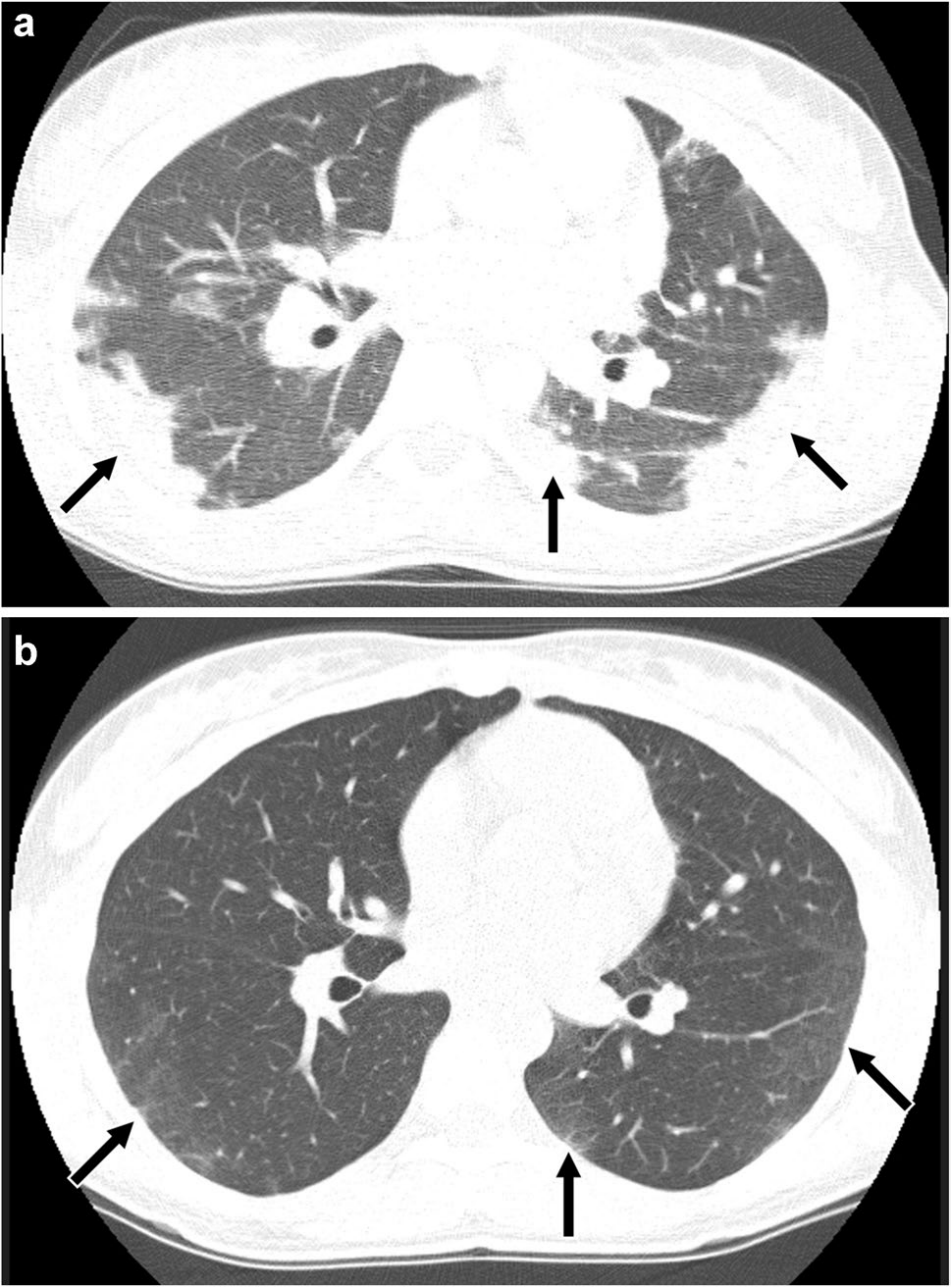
An 18-year-old female with a 3-week history of fever, cough, and dyspnea while receiving minocycline for acne. a Axial CT image with contrast showing bilateral peripheral consolidation (*black arrows*) typical of chronic eosinophilic pneumonia. b Axial image from follow-up CT with contrast obtained after medication discontinuation and 2 weeks of steroid therapy showing substantial improvement, with only mild patchy peripheral ground-glass opacity (*black arrows*) remaining at prior sites of consolidation consistent with minocycline-induced lung disease. (Images reprinted from Caffey’s Pediatric Diagnostic Imaging, 12th Edition, Zucker EJ, Guillerman RP, Fishman MP, Casey AM, Lillehie CW, Lee EY, Diffuse Lung Disease, 594–604, 2013, with permission from Elsevier.)
